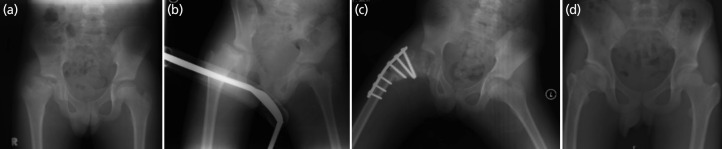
The series of radiographs belongs to patient no.7. (a) The presentation with Herring B and pseudo hinge abduction at the age of 11. (b) Failure of containment with traction. (c) The hip showed good containment after general anaesthesia, released of adductor muscles and FVO. (d) The hip at the age of 16 showed Stulberg III.
